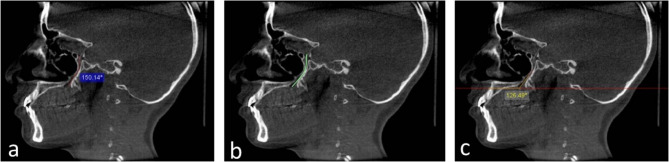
a Sagittal CBCT reformatted image showing the angle formed by the GPC pathway from PPF axis to GPF (red curved line). b Sagittal CBCT reformatted image showing GPC pathway type (b) (green curved line). c Sagittal CBCT reformatted image showing the angle formed between GPC axis and the horizontal plane (yellow curved line)For GPC pathways the following types were developed:aGPC travels in an antero-inferior direction through its whole pathway.bGPC first travels in an inferior direction, then changes its path into an anterior-inferior direction (Fig. 1b).cGPC first travels in a posterior-inferior direction, then then changes its path into an anterior-inferior direction.dGPC first travels in a posterior-inferior direction, then in an inferior direction.eOtherGPC axis-horizontal plane angle (angle formed between a theoretical line perpendicular 146 to the long axis of the body, and the axis of the GPC).
